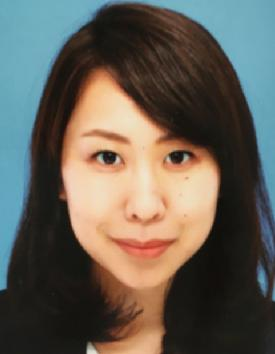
Akiko Masumoto obtained her license to practice medicine at Osaka University, Japan. After completing residency at the Kobe City Medical Center General Hospital, she enrolled as a structural heart disease fellow in cardiovascular medicine at the Hyogo Prefectural Harima-Himeji General Medical Center, where she works currently. She specializes in structural heart diseases and echocardiography.
